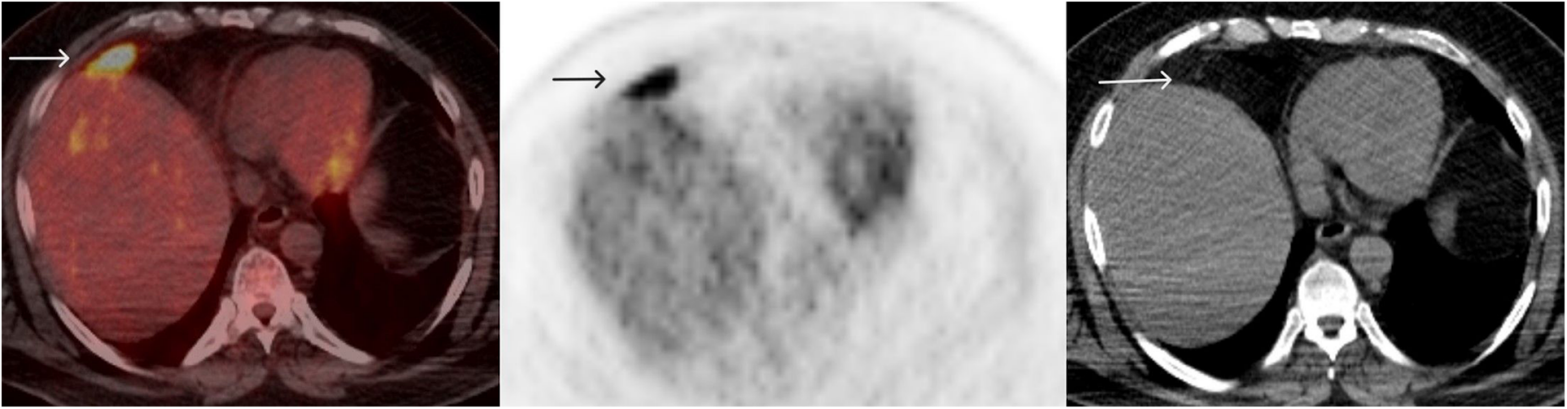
FDG PET/CT December 2018: after 12 cycles of immunotherapy, FDG uptake in the perihepatic lesion increased to SUV max 7.8 (background hepatic SUV max 2.8).
